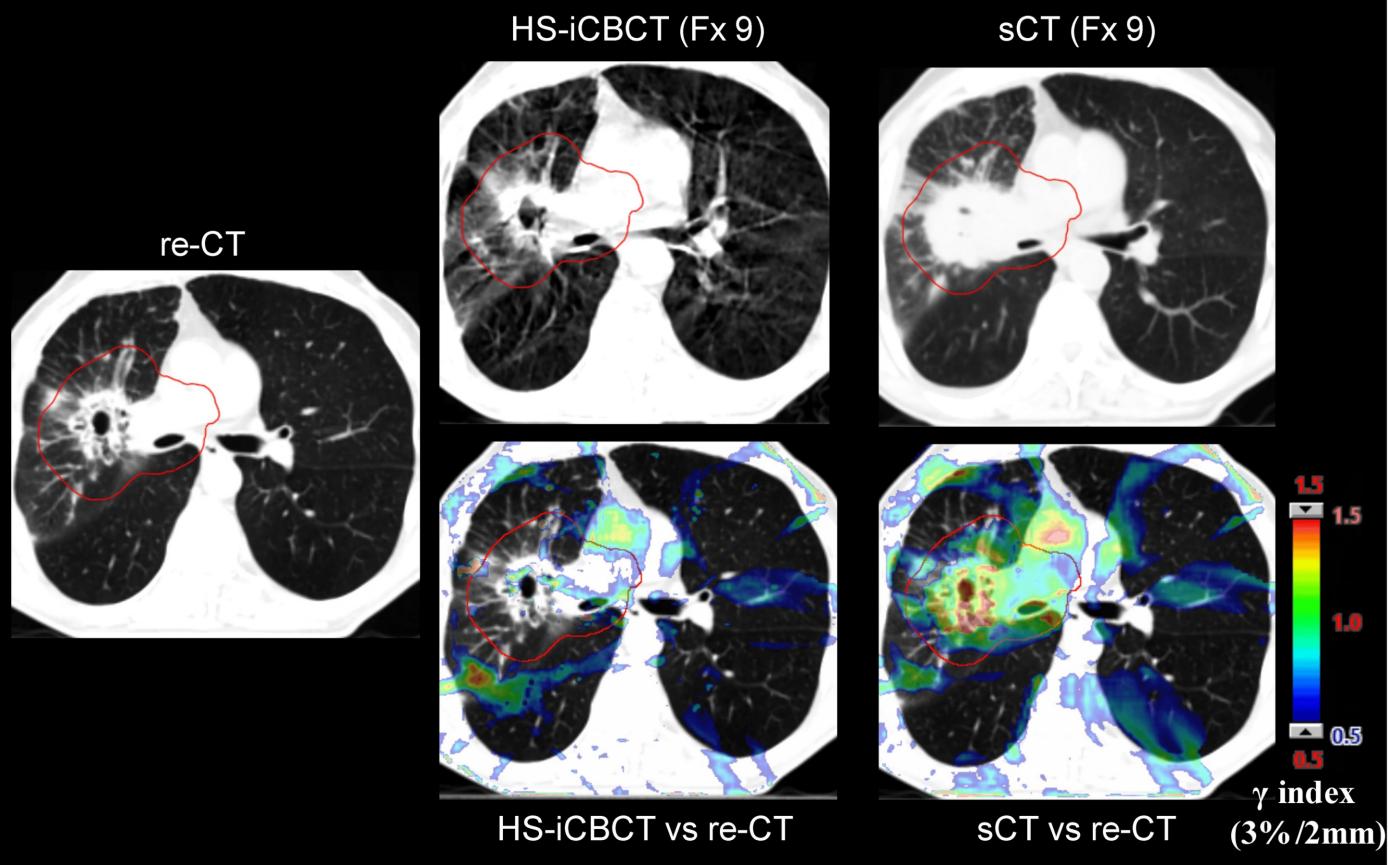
Images from the re-CT, along with HS-iCBCT and sCT at fraction 9, are presented. Using re-CT as the reference, the gamma distributions of HS-iCBCT and sCT with a criterion of 3%/2 mm are shown on re-CT. HS-iCBCT: HyperSight iterative cone-beam computed tomography; sCT: Synthetic computed tomography; Fx: Fraction
